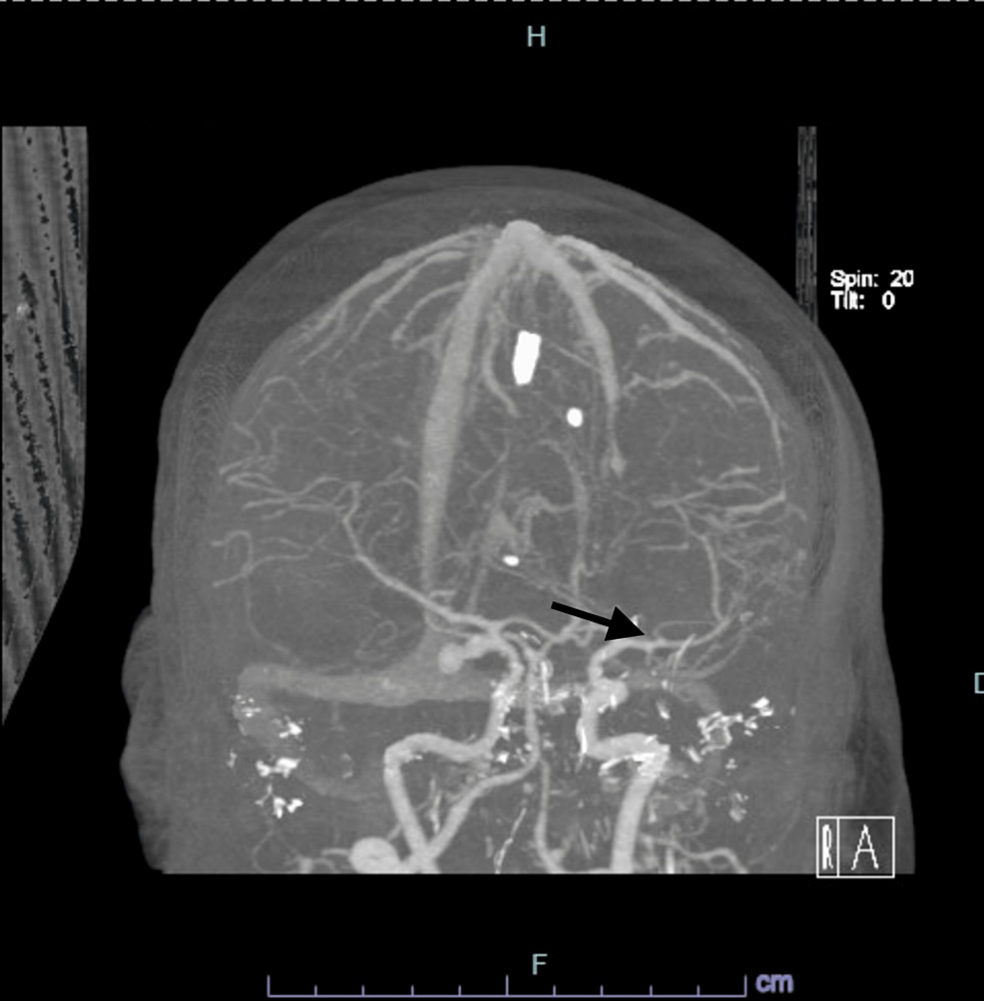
Left Posterior Communicating Artery Aneurysm Computed tomography angiography (CTA) brain showing evidence of a small aneurysm affecting the origin of the left posterior communicating artery (arrow).
